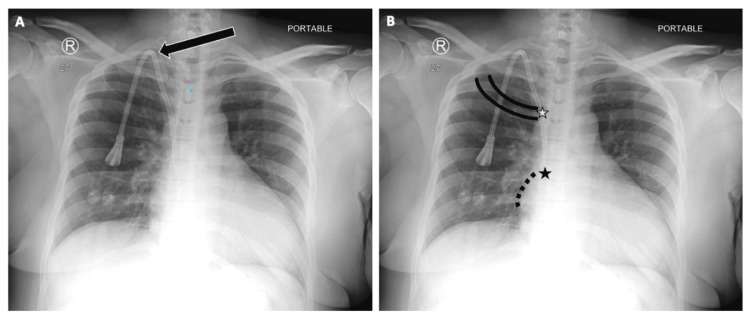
Chest Radiographs Demonstrating Proper Central Venous Catheter (CVC) Placement and Key Anatomical Landmarks Reference chest radiographs of upper extremity CVC placement.
